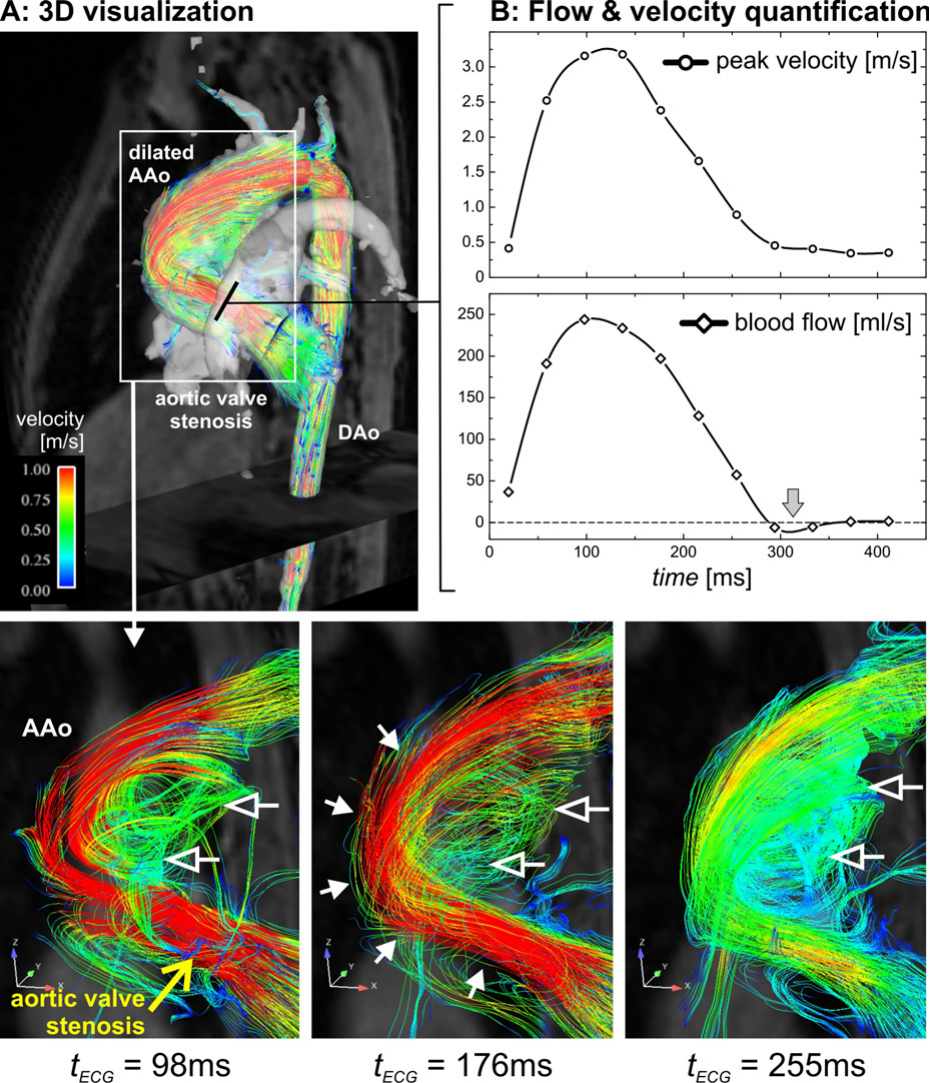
3D flow visualization (A) and flow quantification (B) in a nine year old pediatric patient with aortic valve stenosis (aortic valve area = 1.2 cm^2^) and dilation of the ascending aorta (maximum diameter = 33 mm). Echocardiography demonstrated normal global cardiac function (ejection fraction EF = 72%) but substantial flow acceleration (peak velocity = 2.8 m/s) and an elevated pressure gradient (maximum pressure = 38 mmHg) at the level of the aortic valve. These findings were confirmed by retrospective quantitative analysis of the 4D PC-MRI data in an analysis plane above the aortic valve which revealed high systolic peak velocities (peak velocity = 3.2 m/s, max pressure gradient = 41 mmHg) but only very mild diastolic retrograde flow (gray arrow). 3D flow visualization using streamlines showed localized flow acceleration along the outer wall of the ascending aorta (solid white arrows) which developed into a vortex flow pattern (open arrows) occupying the shape of the aneurysm (AAo: ascending aorta, DAo: descending aorta).
